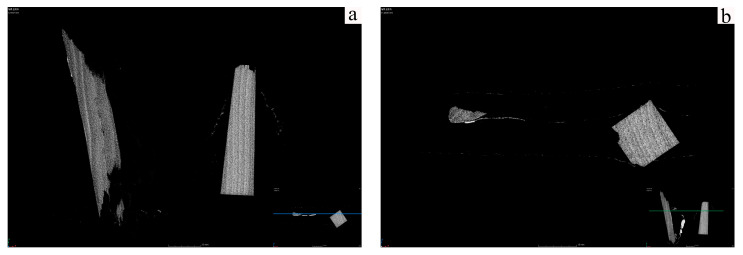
CT images of sample (a) transverse section (The blue line indicates the position of the CT slice), (b) longitudinal section (the green line indicates the position of the CT slice).
